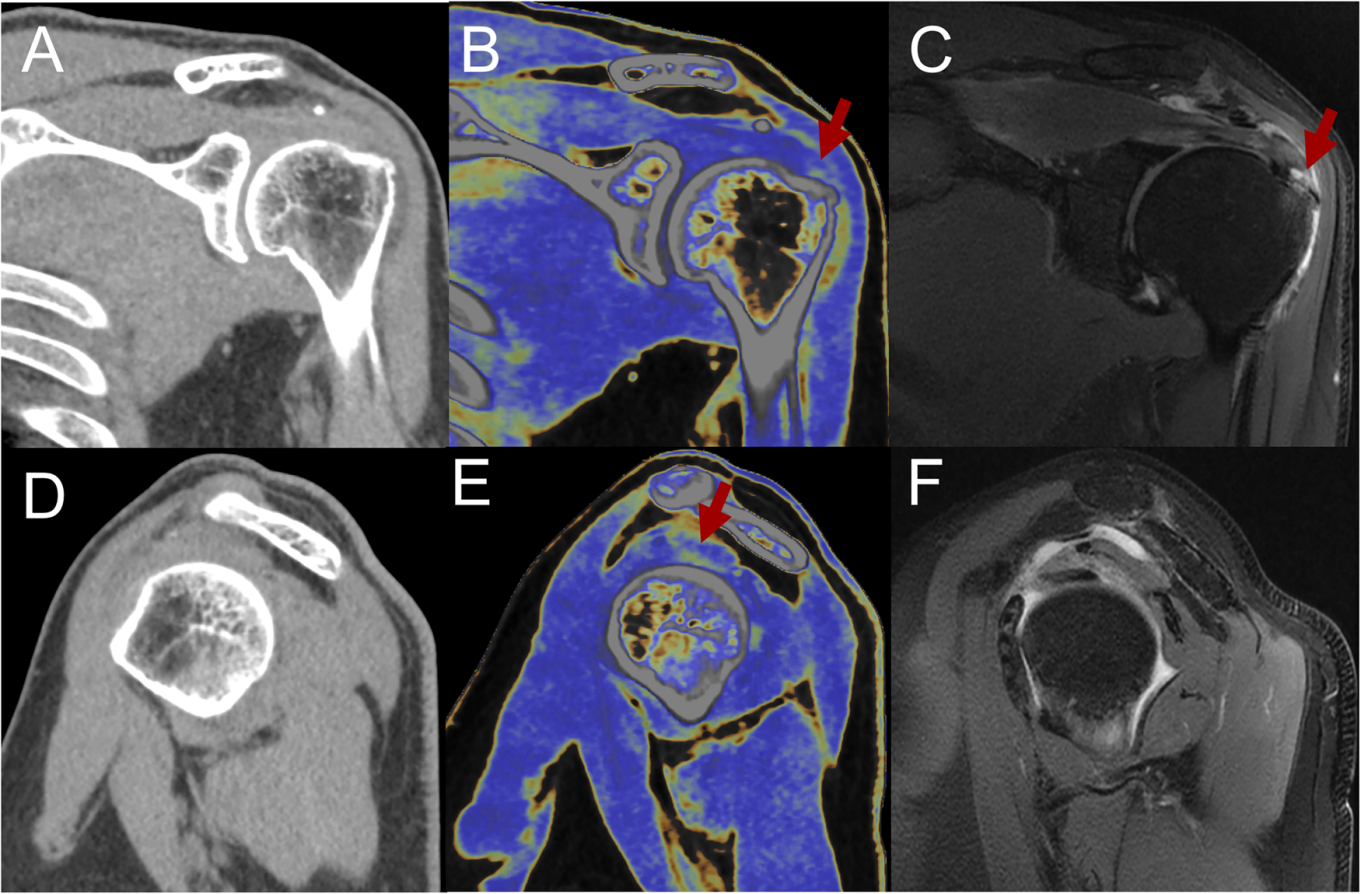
Images for a 60-year-old man with left shoulder pain and limited motion for several months. A, D At standard CT, the supraspinatus tendon partial tear was initially not visible on gray-scale reconstruction. B, E Supraspinatus tendon partial tears were detected after MMD reconstruction images were optimized for tendon analysis. C, F Noncontrast MRI in oblique coronal and oblique sagittal views shows supraspinatus tendon partial tears on fat-suppressed proton density-weighted images (red arrow). MMD, multi-material decomposition
